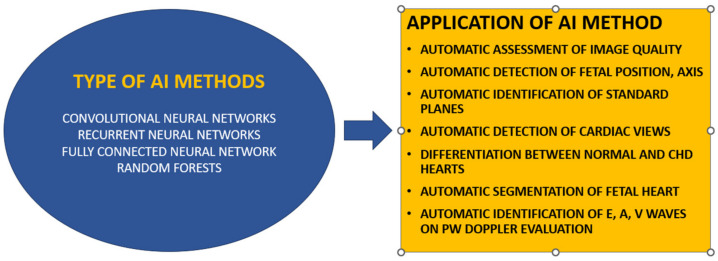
Type of AI methods used to obtain information in fetal echocardiography using DL models.
